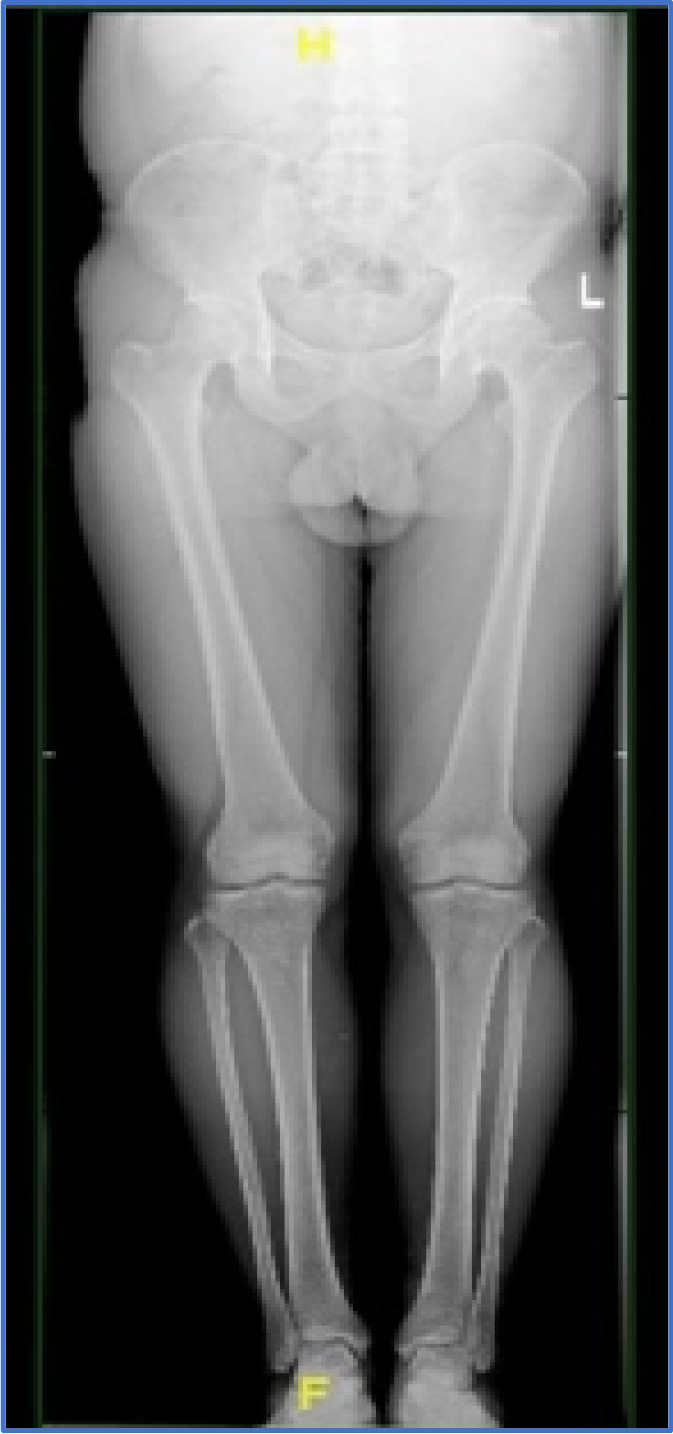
Normal mechanic alignment of right lower limb in standing full‐length radiograph.
